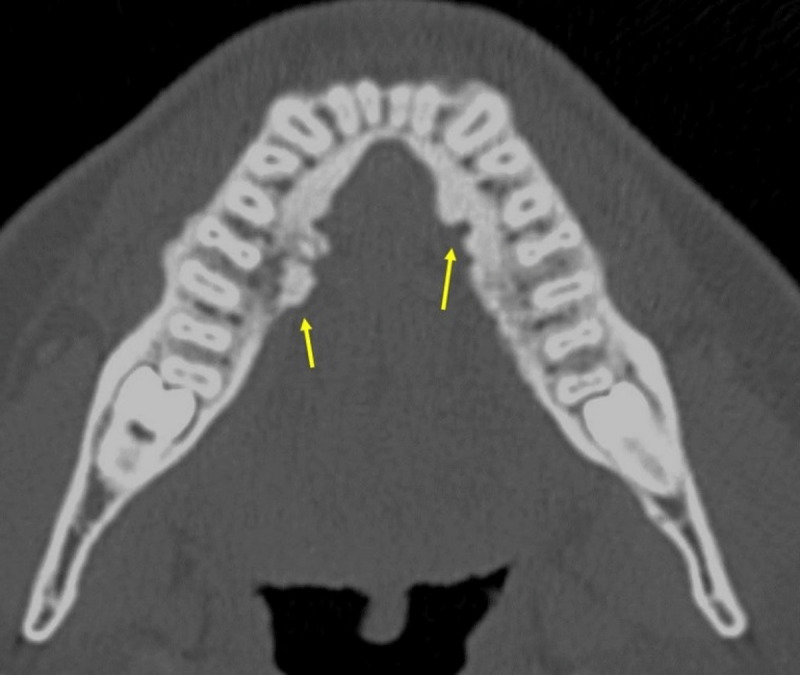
Axial CT showing torus mandibularis.
